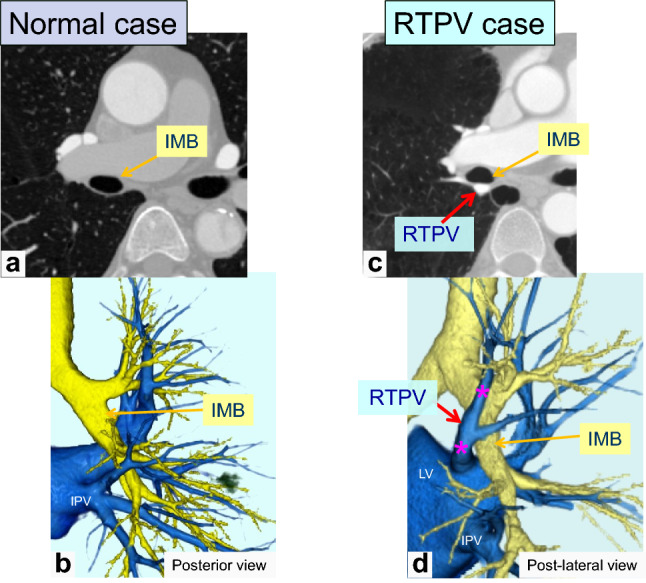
Thin-section and 3D-CTA images of a normal case and a case of right top pulmonary vein (RTPV). Thin-section CT (a) and 3D-CTA (b) images showing the normal pattern of the pulmonary vein. Thin-section CT (c) and 3D-CTA (d) image showing the RTPV
